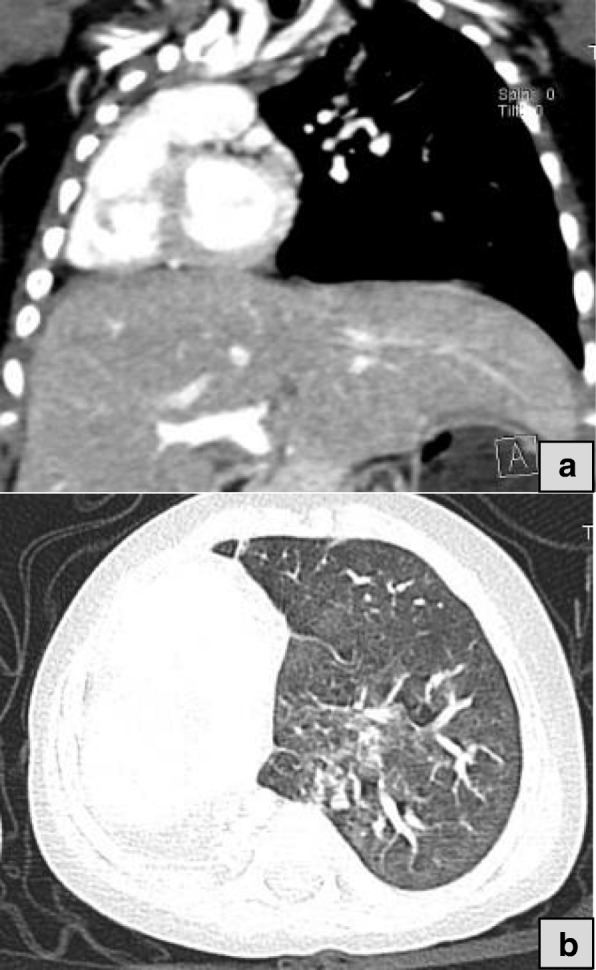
a Coronal CT image in mediastinal window shows complete absent of right lung, bronchus and vessels with complete heart and mediastinal shift into the right hemithorax. Fig. 1:b CT axial image in lung window demonstrates absence of right lung parenchyma with its vasculature and location of heart and great vessels in the right side. (Four months’ boy)
